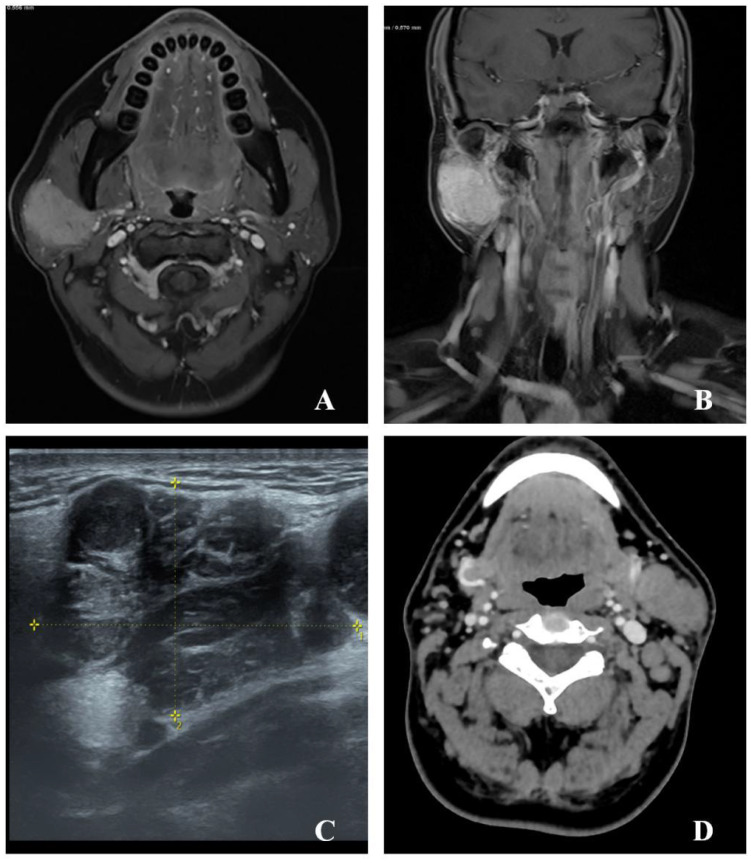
Lymphoepithelial carcinoma of salivary glands. MRI, Axial contrast-enhanced T1-weighted imaging (A) and coronal contrast-enhanced T1-weighted imaging (B) demonstrated a mass with relatively clear boundaries and heterogeneous mild-to-moderate enhancement. Color Doppler, A hypoechoic mass was detected in the lower pole of the left parotid gland, featuring clear boundaries, an irregular and lobulated shape, a relatively dense internal structure, and linear hyperechoic foci (C). CT, An irregularly shaped mass with heterogeneous enhancement was observed in the left parotid gland region (D).
